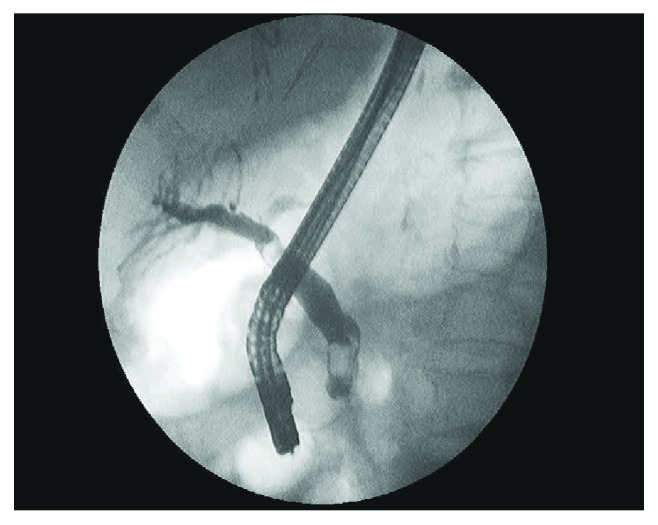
Common bile duct stones on X-ray fluoroscopy.
